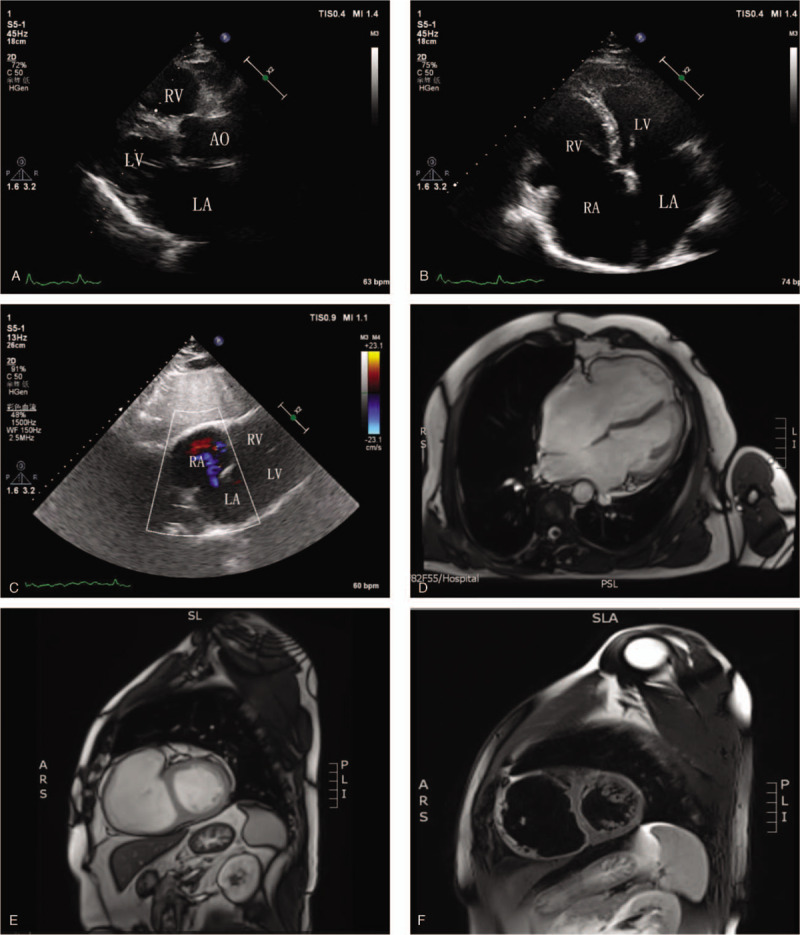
The echocardiography of the patient. (A) The parasternal long axis view; (B) The apical 4 chamber view; (C) The subxiphoid 4 chamber view. The cardiac magnetic resonance imaging of the patient which shows typical change of ARVC (D, E, F). ARVC = arrhythmogenic right ventricular cardiomyopathy.
